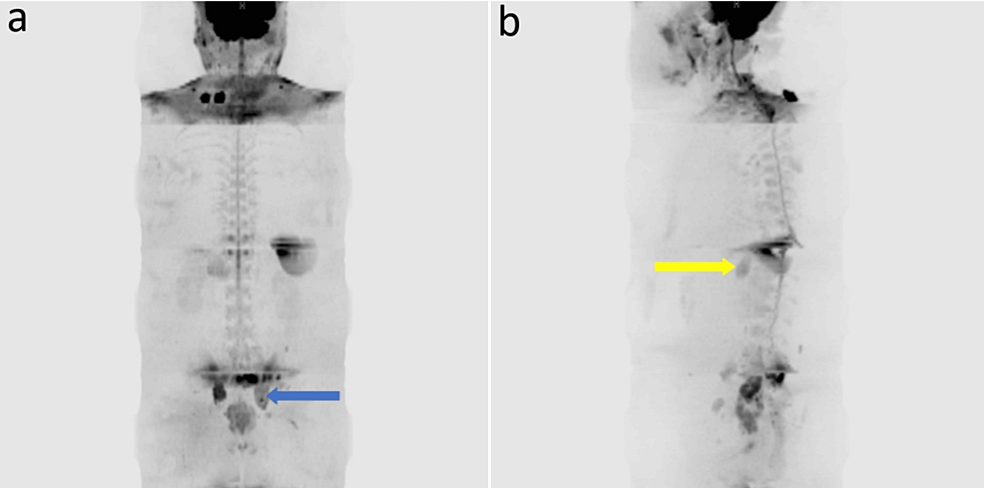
WB-DWI/MRI with coronal MIP reconstruction Whole-body diffusion-weighted MRI (WB-DWI/MRI) with coronal maximum intensity projection (MIP) reconstruction showing metastatic prostate cancer, with (a) pelvic bone metastasis (blue arrow) and (b) retroperitoneal metastases (yellow arrow).
